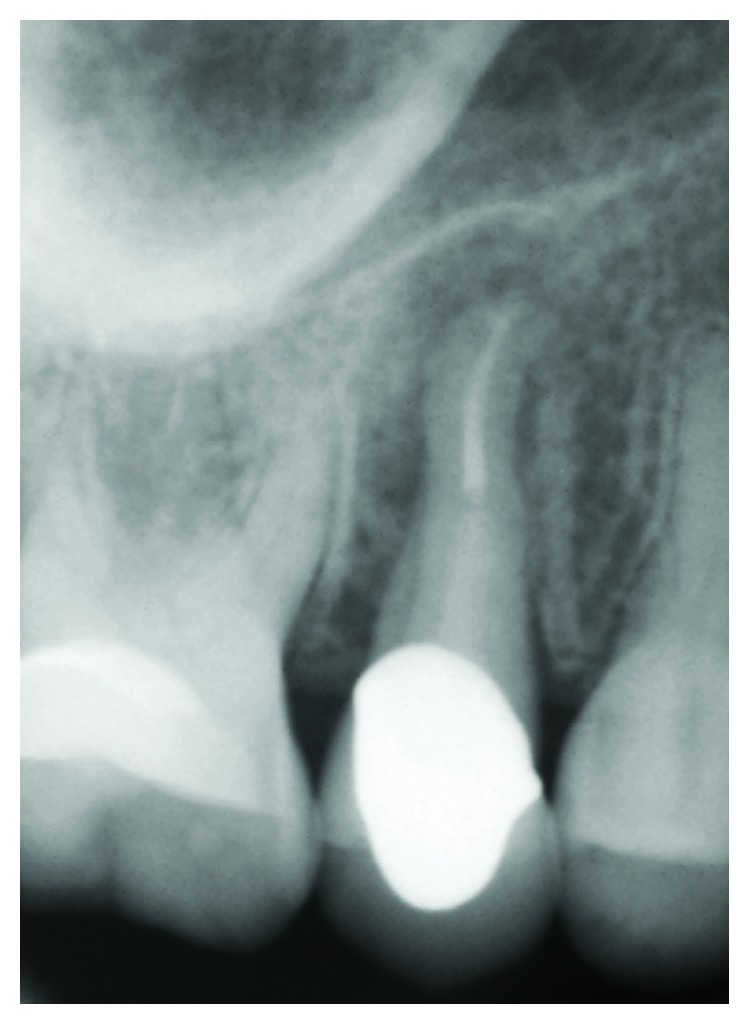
Development of clinical symptoms 9 years after crown delivery: the radiograph revealed a periapical radiolucency, with widening of periodontal ligament/lamina dura modifications.
